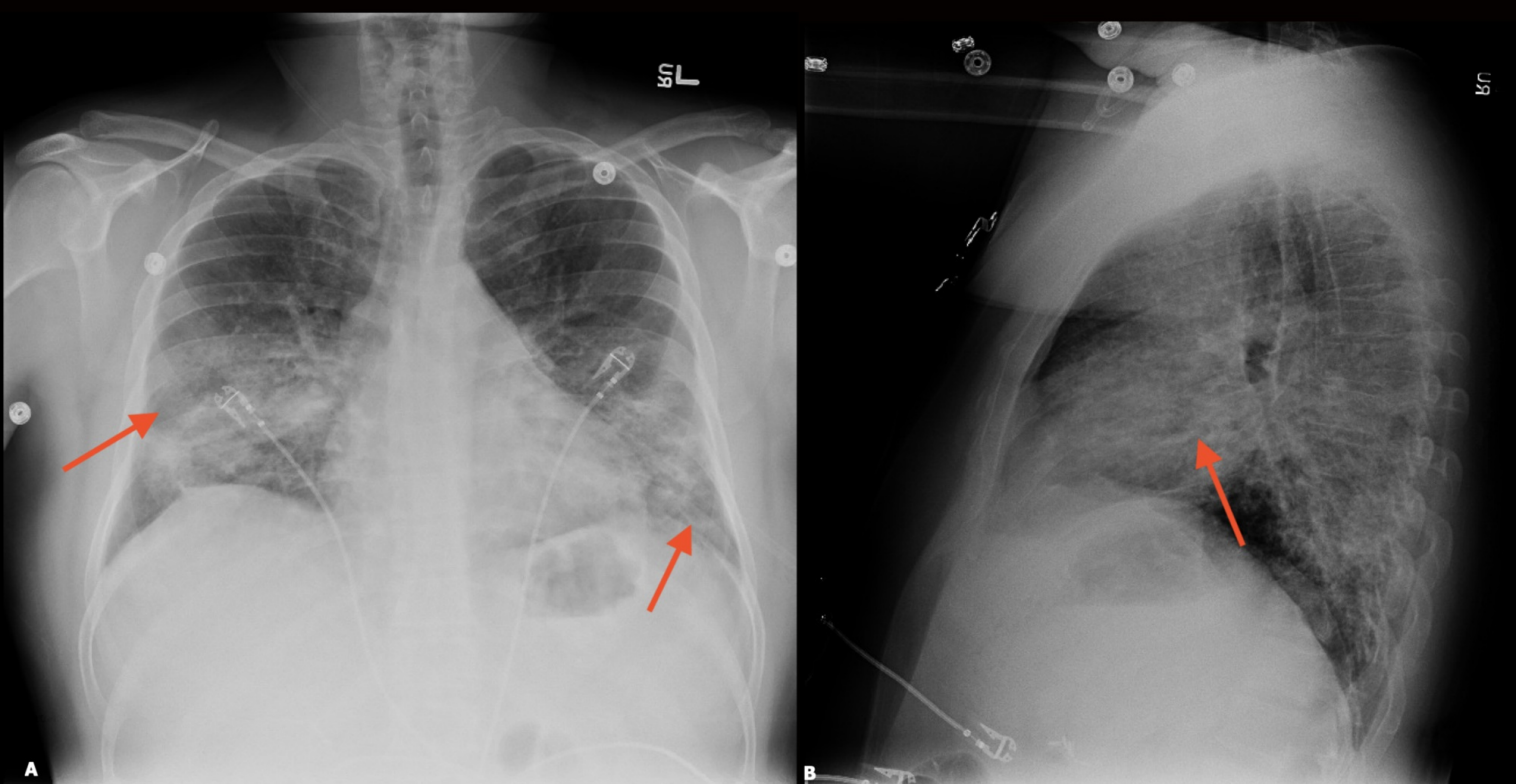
Chest x-ray after the initiation of steroids Interval improvement was seen in bilateral airspace opacities (red arrows). A) Postero-anterior view; B) lateral view
